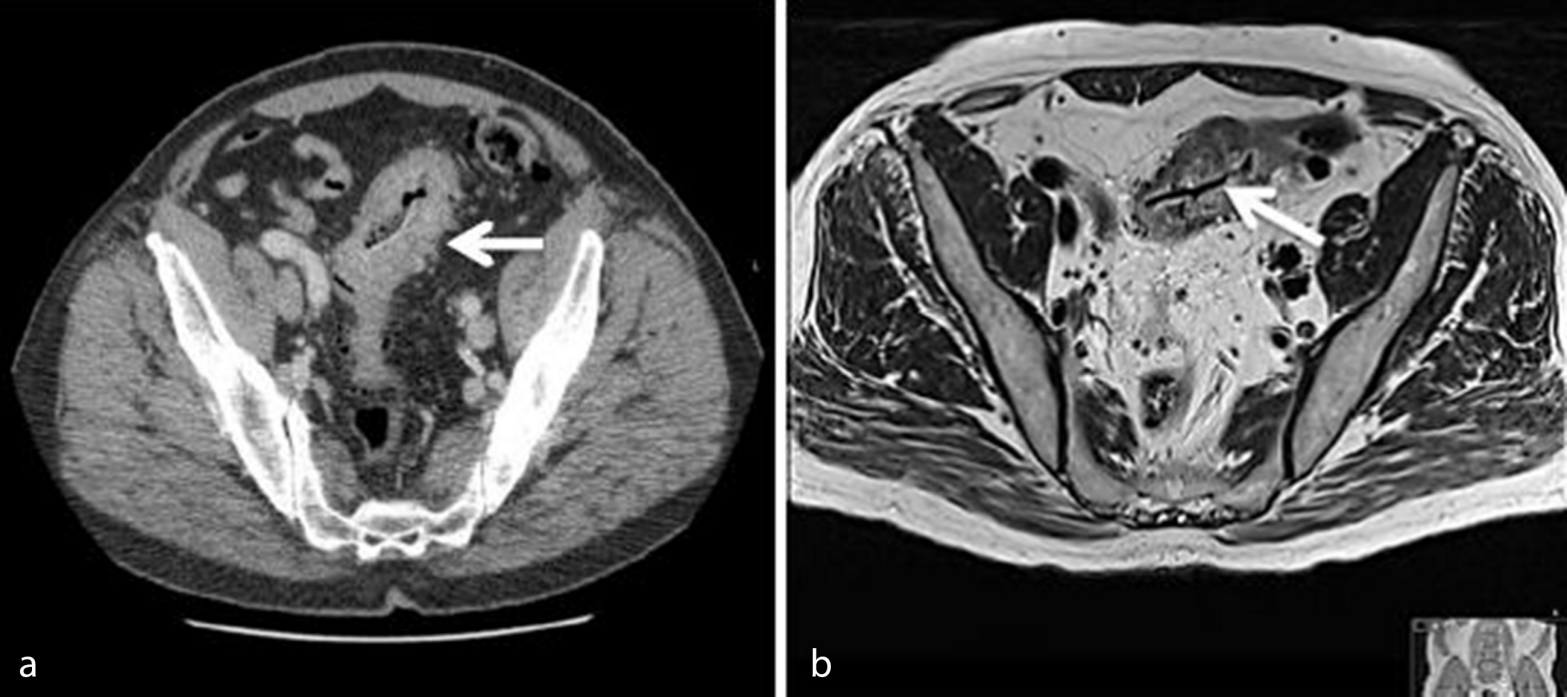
(a) CT axial slice through the pelvis showing the thickened distal sigmoid colon with multiple diverticulae (white arrow). The linear hyperdense line on the intraluminal surface represents the poorly demarcated dental prosthesis. (b) MRI axial slice corresponding to the CT image showing an intraluminal low signal, well-defined linear structure corresponding to the dental prosthesis.
